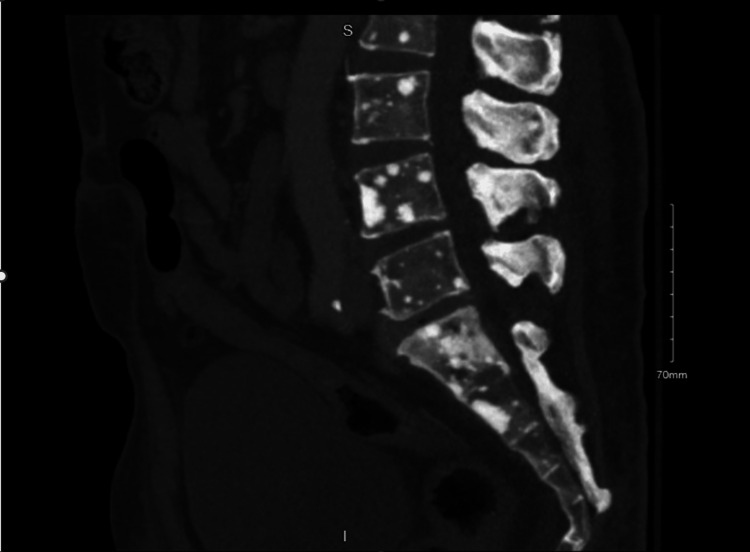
Lateral CT abdomen/pelvis CT: computed tomography
